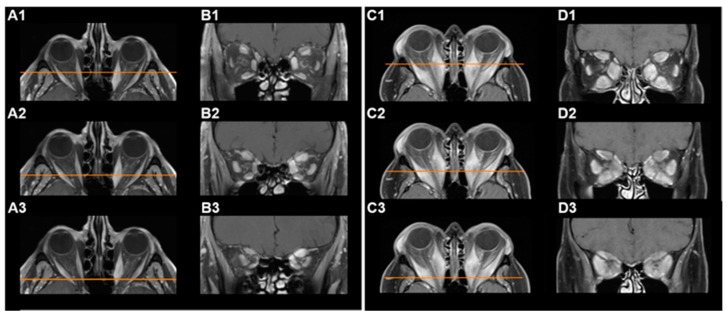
Magnetic resonance imaging (MRI) demonstrating not-increased or increased orbital muscle volume. (A,B): T1-weighted, contrast-enhanced MRI scans of the orbits of a bilaterally affected GO-patient with not-increased orbital muscle volume prior to decompressive surgery; axial images (A1–A3) with the orange line indicating the position of each corresponding coronal (B1–B3) image retrobulbar (A1,B1), at the orbital apex (A3,B3) and in between (A2,B2). (C,D): T1-weighted, contrast-enhanced MRI scans of the orbits of a bilaterally affected GO-patient with increased orbital muscle volume prior to decompressive surgery; axial images (C1–C3) with the orange line indicating the position of each corresponding coronal (D1–D3) image retrobulbar (C1,D1), at the orbital apex (C3,D3) and in between (C2,D2).
